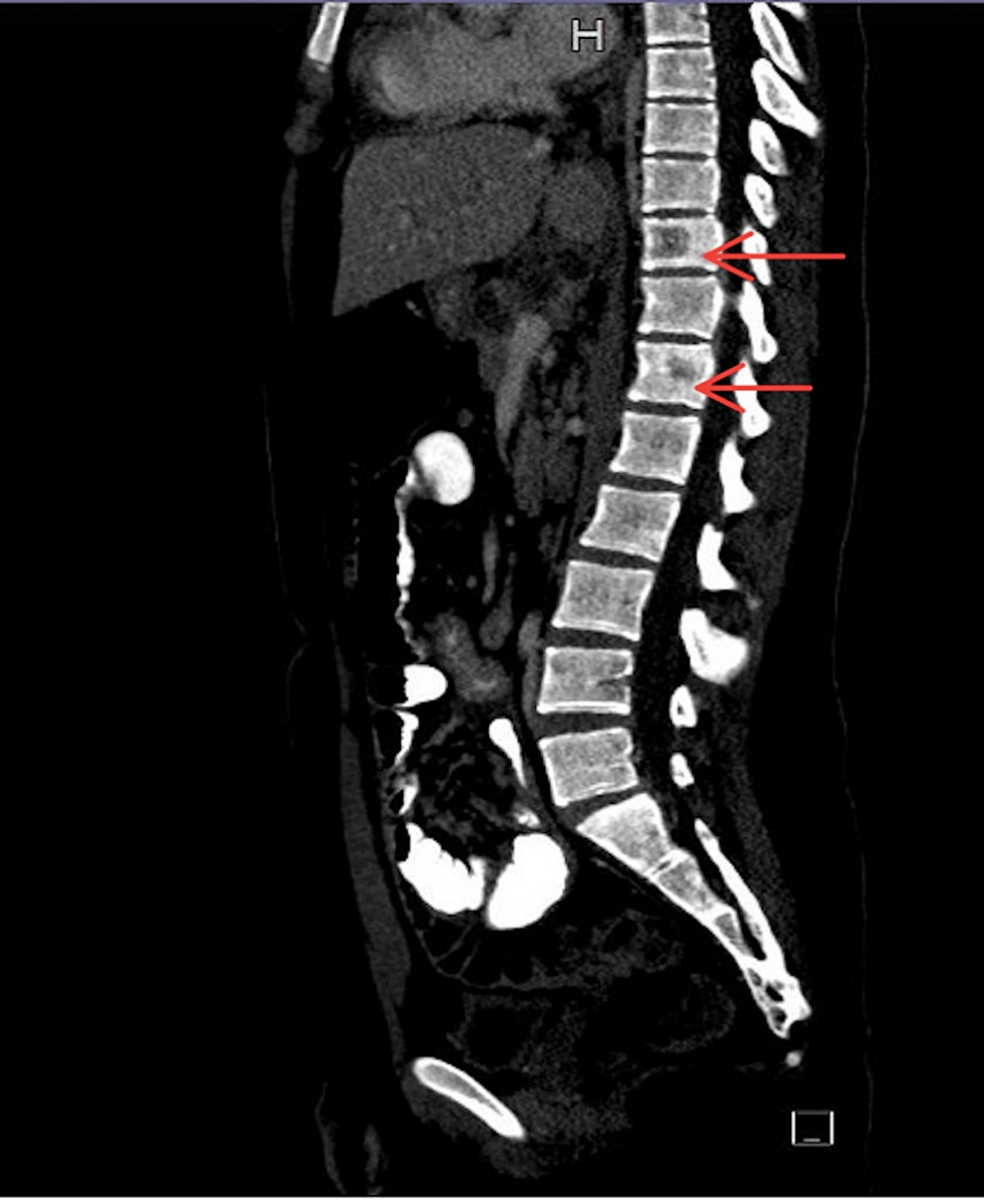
CT chest with IV contrast showed bony lytic lesions on T10 and T12 IV: Intravenous
